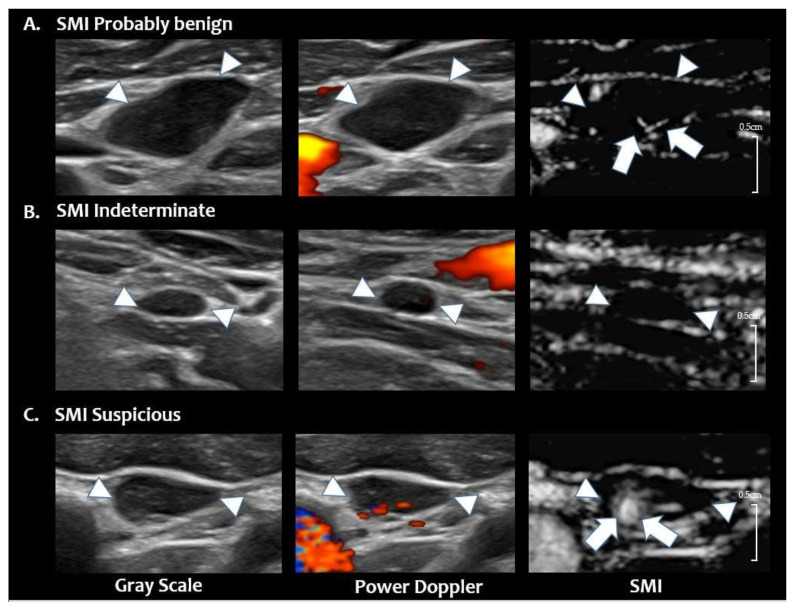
Reclassified LN categories of SMI. (A) SMI probably benign LN. Grey scale and PDUS images show a hypoechoic LN with loss of hilum and hilar vascularity in the left neck level 3. On SMI, central hilar vascularity is noted (arrows), and this LN is classified as ‘SMI probably benign’ (final diagnosis-benign on FNA-Tg) (B) SMI indeterminate LN. Grey scale and PDUS show a small LN in right neck level 4 with loss of hilum and hilar vascularity. On SMI, no additional vascular signals are noted; therefore, this LN is classified as ‘SMI indeterminate’ (final diagnosis-benign on FNA-Tg). (C) SMI suspicious LN. Grey scale and PDUS show a hypoechoic LN with eccentric cortical thickening and displaced hilar vascularity in left neck level 3. On SMI, abnormal peripheral vascularity is noted at the medial portion of the LN (arrows, final diagnosis-metastatic PTC on CNB). The arrowheads in figures A, B, C indicates the margin of the LN. PTC = papillary thyroid carcinoma, US = ultrasound, LN = lymph node, PDUS = power Doppler ultrasound, SMI = superb microvascular imaging, FNA = fine needle aspiration, CNB = core needle biopsy, Tg = thyroglobulin.
